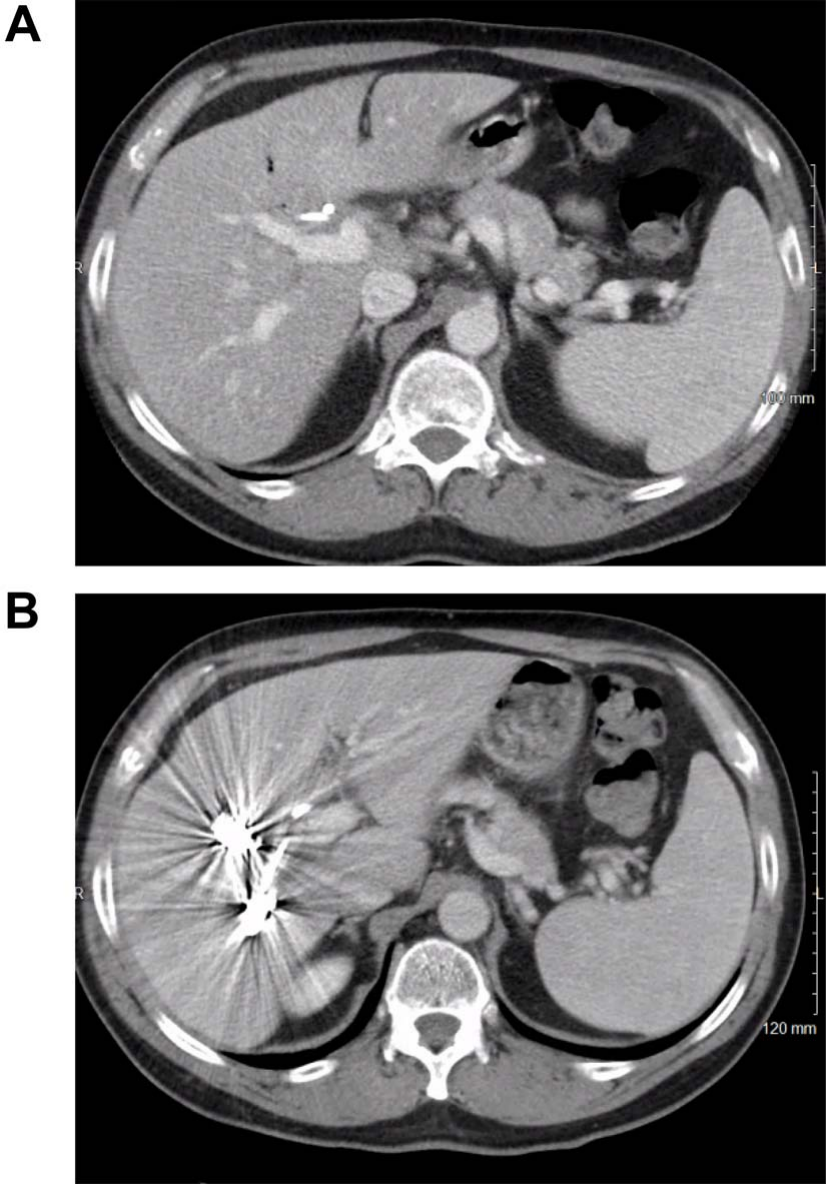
CT scan image of the liver: (A) small left lobe prior to portal vein embolization; (B) left lobe hypertrophy after right portal vein embolization.
